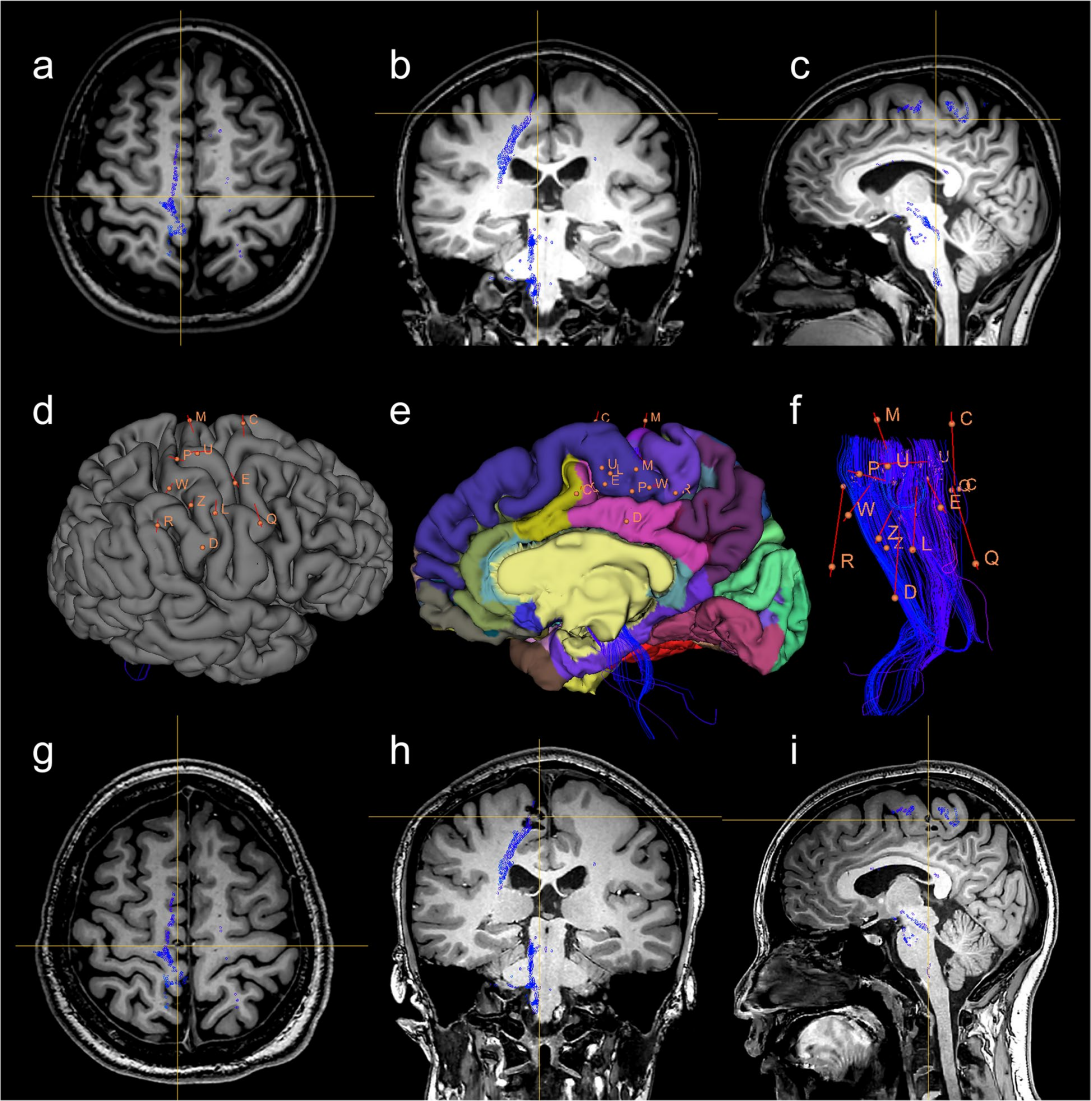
Preoperative and postoperative image evaluation (Patient 1). a–c Preoperative MRI T1 images showing the location of thermo-lesions in the right paracentral lobule in the axial, coronal and sagittal plane fused with the corticospinal tract of the lower limb. d Lateral view of spatial relationship between electrodes and the three-dimensional cerebral model. e Medial view of spatial relationship between the targets of electrodes and the three-dimensional cerebral model, with cortical parcellation marked by different colors. f Spatial relationship between trajectories of electrodes and right corticospinal tract of the lower limb. g–i Postoperative MRI T1 showing the range of thermo-lesions in the axial, coronal and sagittal plane fused with the right corticospinal tract of the lower limb. Blue streamlines in (a–c) and (g–i), and blue lines in (d–f) are the right corticospinal tract of the lower limb. Crossing point of yellow lines in (a–c) and (g–i) is the location of thermo-lesions. Orange lines in (d–f) present electrodes with their corresponding letters
